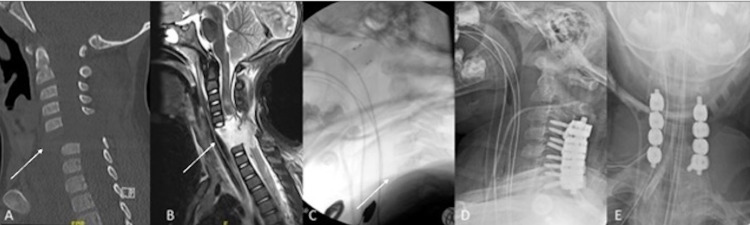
Pre- and postoperative imaging. A: Preoperative CT demonstrating C5/C6 fracture-dislocation (arrow); B: preoperative MRI redemonstrating C5/C6 fracture-dislocation and complete transection of the spinal cord (arrow); C: intraprocedural halo reduction fluoroscopy demonstrating realignment of vertebral bodies (arrow); D: postoperative lateral X-ray; E: postoperative anteroposterior X-ray
